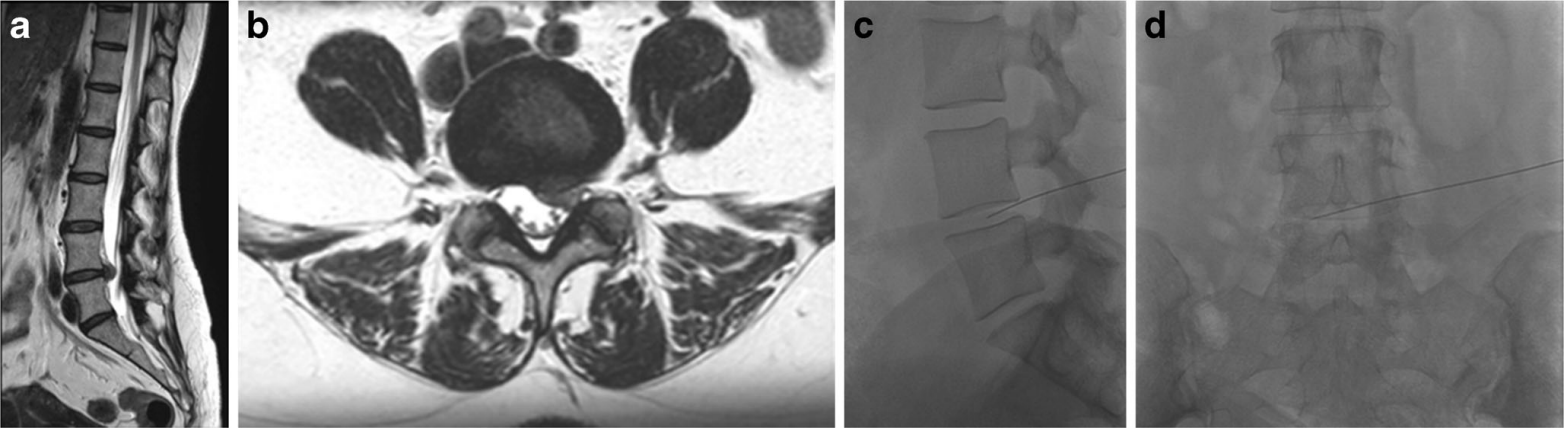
A 59-year-old women complaining of left lumbosciatica of 6-month duration and treated with ozone chemonucleolysis. a Sagittal MRI T2-weighted image showing degenerative disc changes and a large extruded disc herniation at the level of L4–5, compressing the dural sac. b The corresponding axial MR T2-weighted image shows the disc herniation located at a left para-median site, obliterating the lateral canal recess with compression of the dural sac and traversing L5 nerve roots. c, d Lateral and anteroposterior fluoroscopic images showing the needle inside the disc center
